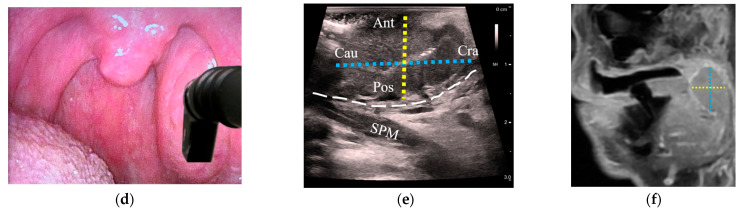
Comparison of tumor diameters between transoral US and corresponding MRI images of a stage-T2 palatine tonsil HPV+ SCC: (a) a patient with an asymmetric left palatine tonsil with an inserted hockey-stick ultrasound transducer placed onto the tonsil in a transverse plane; (b) transverse US reveals a palatine tonsil tumor. Tumor diameters are represented from medial (Med) to lateral (Lat) with a red dotted line and from anterior (Ant) to posterior (Pos) with a yellow dotted line; (c) axial MRI with the corresponding diameters as in (b, red and yellow dotted lines); (d) transducer oriented in the sagittal plane; (e) sagittal US with the cranial (Cra) to caudal (Cau) diameter added (blue dotted line) along with Ant-Pos (yellow dotted line); (f) sagittal MRI with the corresponding diameters as in (e, blue and yellow dotted lines). US: ultrasound; MRI: magnetic resonance imaging; HPV+ SCC: human papillomavirus-positive squamous cell carcinoma; ICA: internal carotid artery; ECA: external carotid artery; MPM: medial pterygoid muscle; SPM: stylopharyngeus muscle; white dashed line: constrictor muscle; Ant: anterior; Pos: posterior; Med: medial; Lat: lateral; Cra: cranial; Cau: caudal.
